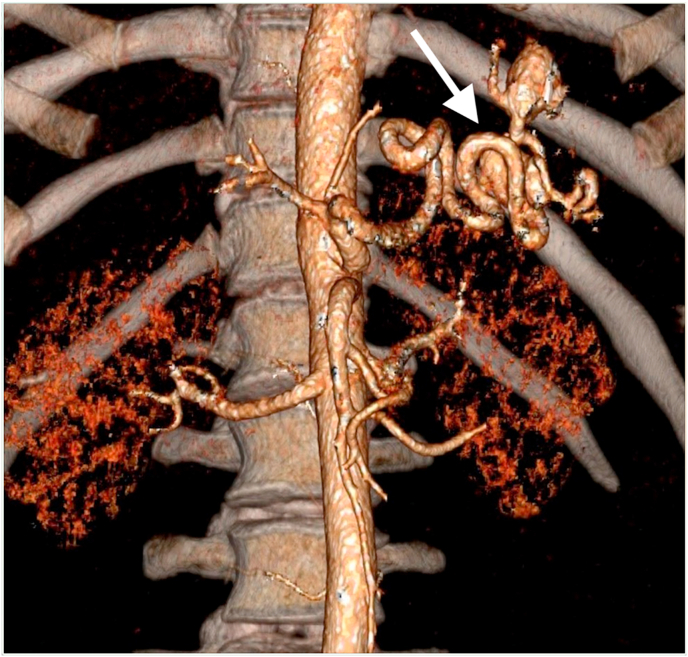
Coronal CT reconstruction. White arrow showing splenic arterial aneurysm.
